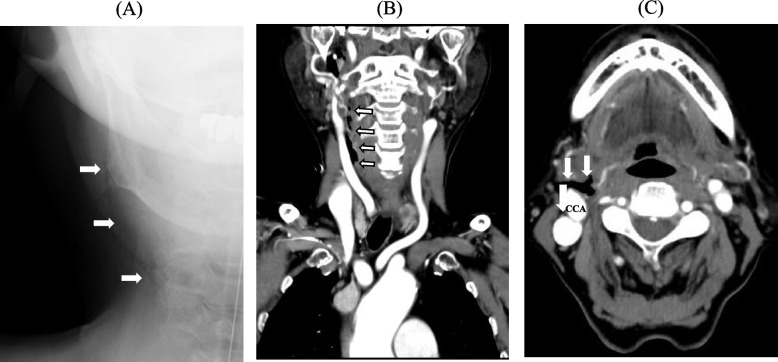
Postoperative chest X-ray (A) and contrast-enhanced computed tomography (B) (C) images. Subcutaneous emphysema was observed in the right side of the neck (A), and it extended up to the right carotid artery (B) (C). CCA, common carotid artery
